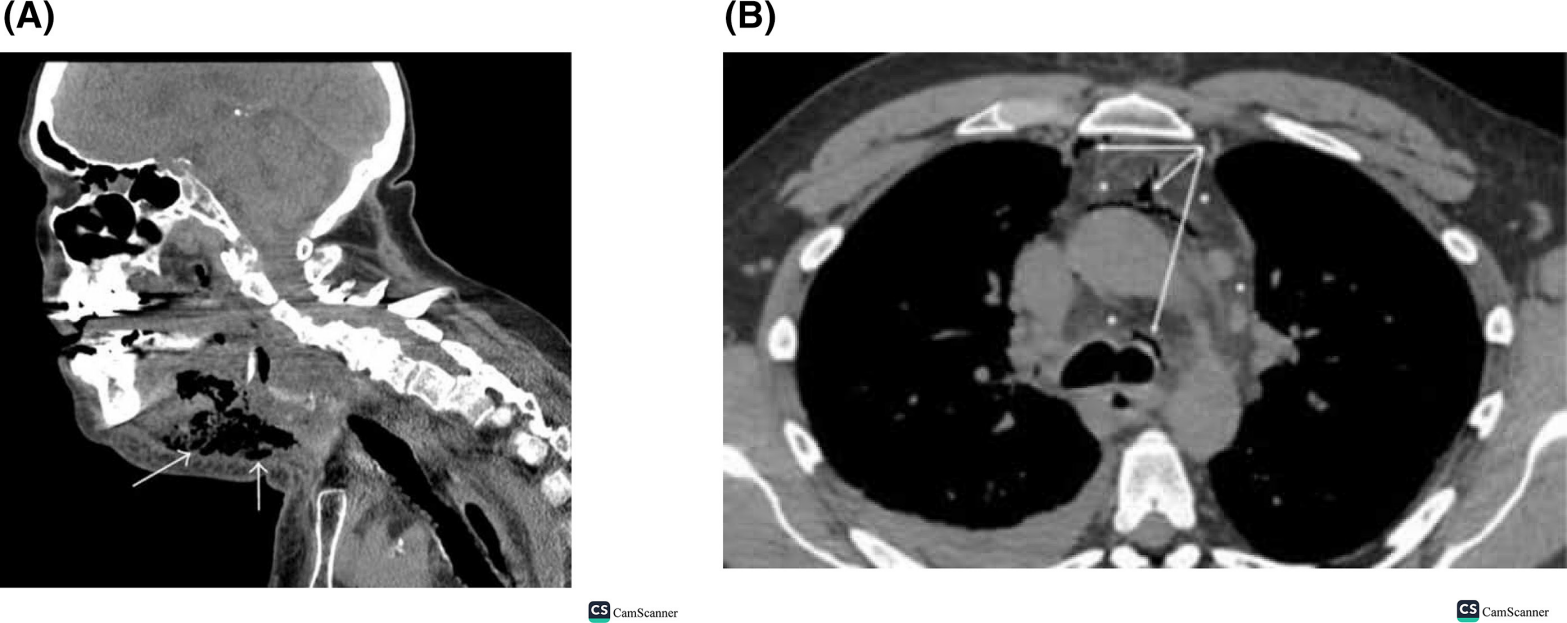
(A) A neck‐CT scan with Contrast. (B) The upper chest with mild pleural effusions. Revealed a pronounced subcutaneous tissue paracardial hypodense lesion and mild pericardial effusion. Midline localized collection detected extending to both submandibular spaces measuring about 5.5 × 12.5 × 9.5 cm with mural enhancement.
